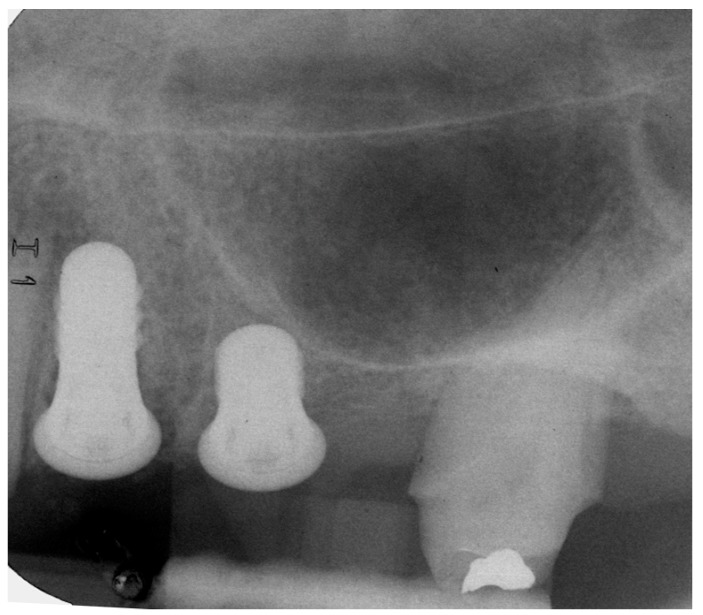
Radiograph of extra short and long implants at implant placement.
